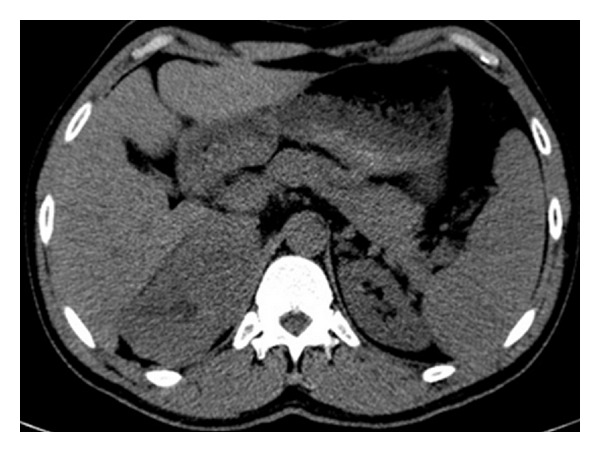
Axial noncontrast CT at the level of adrenal fossa shows a well-capsulated right adrenal solid lesion measured (11 × 6 cm) of relative isodense pattern in comparison to renal parenchyma with central hypodense area; the lesion displays 37 HU.
